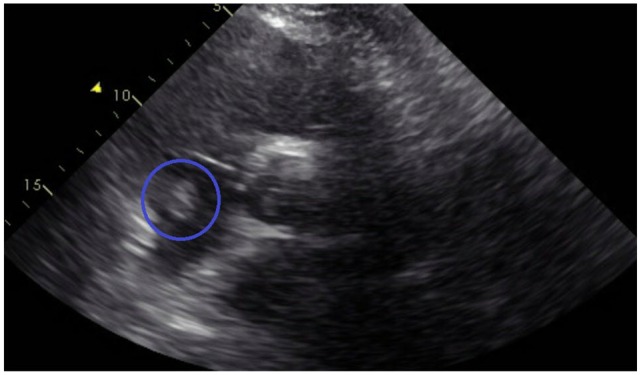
Echocardiogram showing thrombus in the right atrium.
